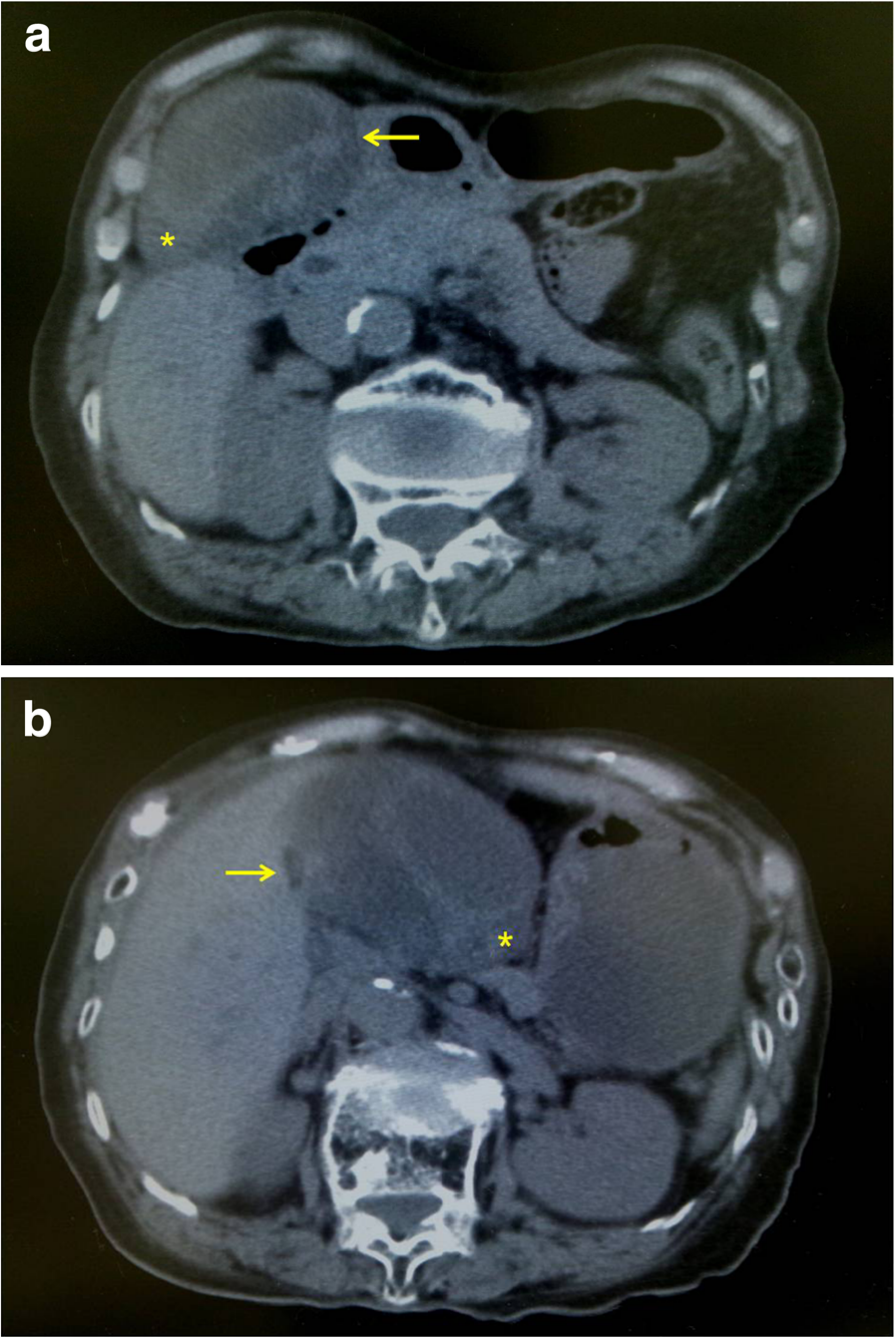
(a) Computed tomography scan of the patient’s abdomen at admission. The gallbladder was enlarged and wall thickened without gallstones, and it was located in its normal anatomical fossa. The arrow shows the cystic duct before rotation. The asterisk shows the gallbladder fundus before rotation. (b) Computed tomography scan of the patient’s abdomen a half day after admission. The gallbladder had rotated approximately 180 degrees and deviated to the midline of the abdomen. It became even more distended and wall thickened. The cystic duct was located on the right side of the gallbladder (arrow). The asterisk shows the gallbladder fundus after rotation.
